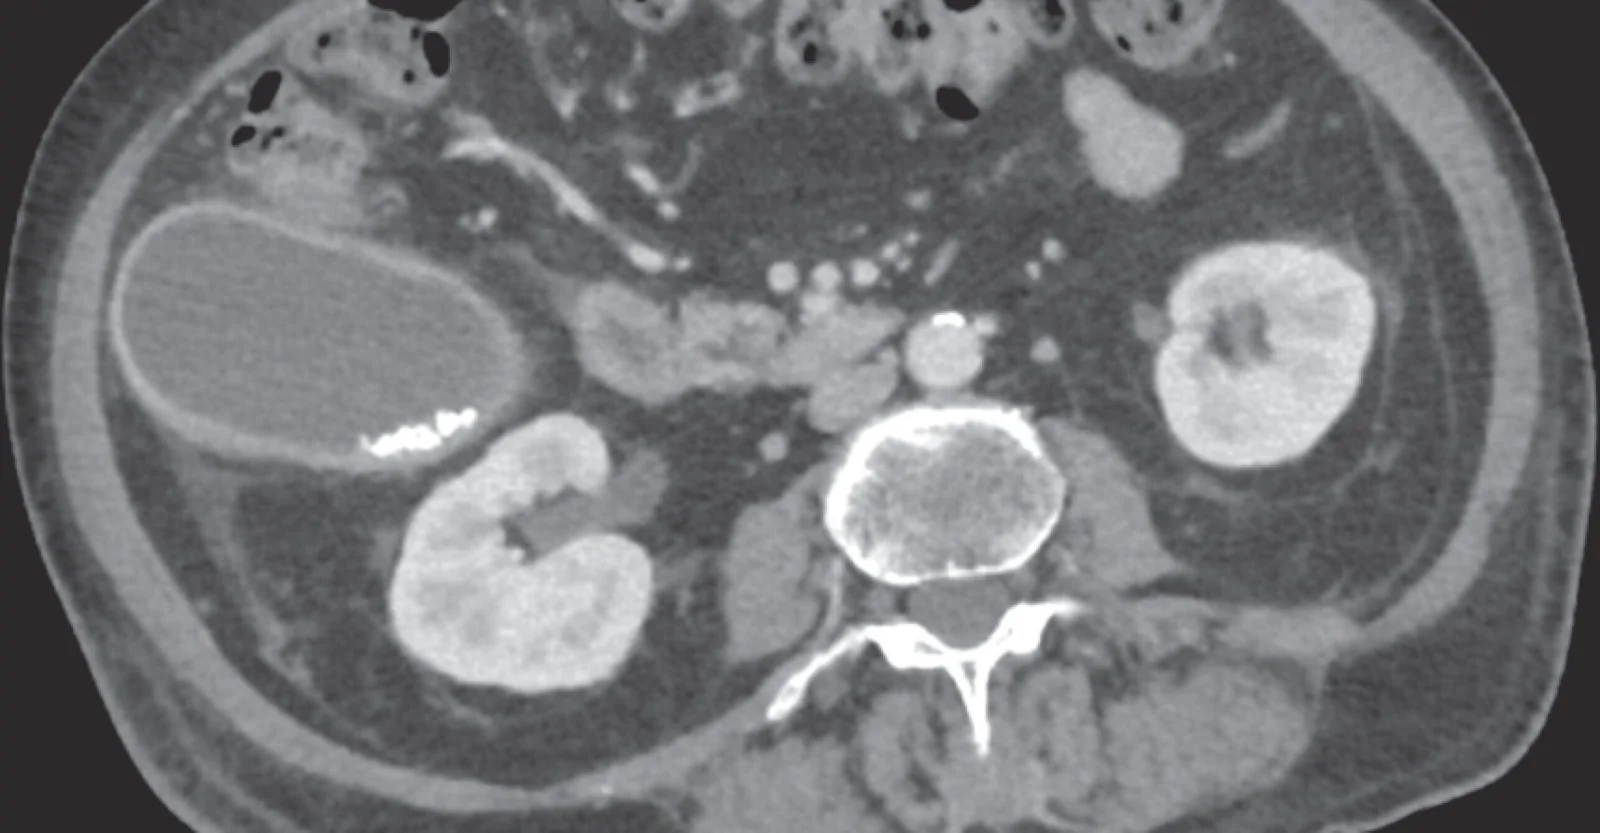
The CT scan showing dilated gallbladder with multiple
